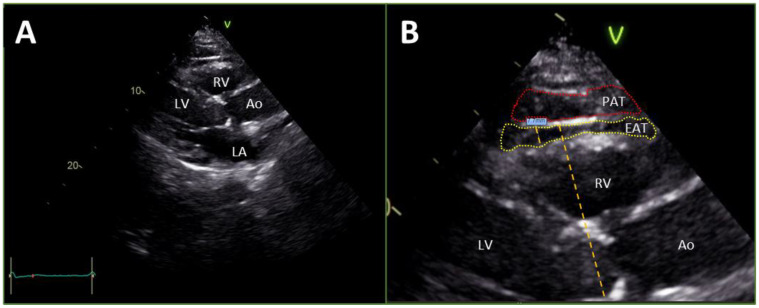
(A) The parasternal long axis view from a transthoracic echocardiogram of the heart at end-systole. Depth is quantified on the right axis in centimeters. (B) [An enlargement of image in A]The measurement of EAT thickness taken perpendicular to the free wall of the RV using the aortic annulus as an anatomical landmark for alignment and orientation (as shown with the orange dashed line). EAT is circumscribed with a yellow dashed line and PAT is circumscribed with a red dashed line. Ao, Aorta; EAT, epicardial adipose tissue; LA, left atrium; LV, left ventricle; PAT, pericardial adipose tissue; RV, right ventricle.
